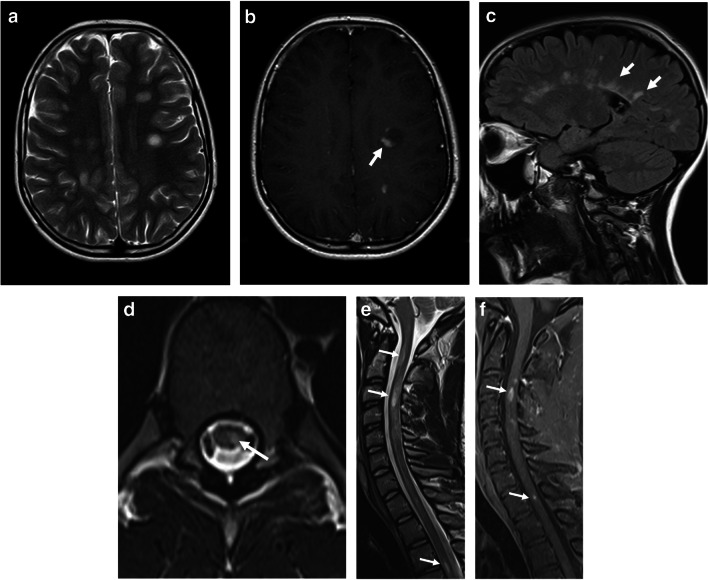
Magnetic resonance images obtained from three different teenagers diagnosed with pediatric-onset multiple sclerosis. a–c Brain involvement in an 11-year-old girl (a, b) and a 15-year-old girl (c). a Axial T2-weighted image shows bilateral, small, well-demarcated ovoid foci of increased T2-signal involving the subcortical and deep fronto-parietal white matter. b Axial contrast-enhanced T1-weighted image shows associated open-ring enhancement (*arrow*). c Sagittal fluid-attenuated inversion recovery image shows multiple high-signal foci radially oriented, perpendicular to the ventricular wall (*arrows*). d–f Spinal involvement in a 15-year-old boy. d Axial T2-weighted image at thoracic level shows a small hyperintense lesion involving the left lateral aspect of the spinal cord (*arrow*). e, f Sagittal T2-weighted (e) and fat-suppressed contrast-enhanced T1-weighted (f) images of the upper spinal cord show short-segment hyperintense T2-lesions (*arrows* in e), associated with nodular enhancement (*arrows* in f)
